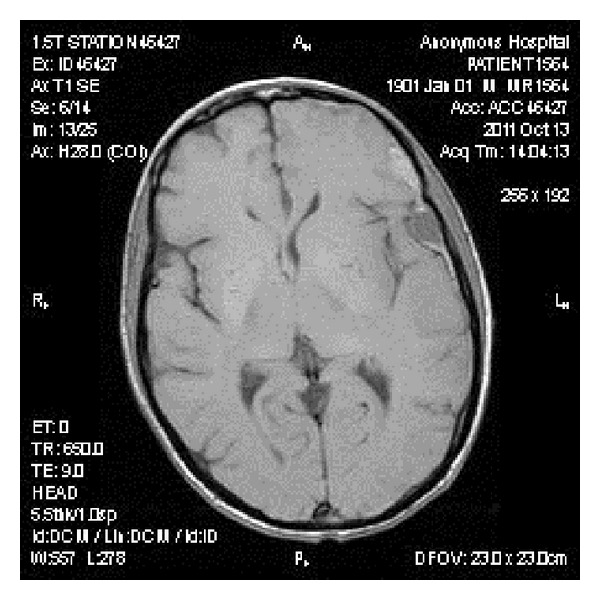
Brain MRI with and without contrast showing a nodular mass reflecting metastatic disease displacing the left inferior frontal gyrus.
